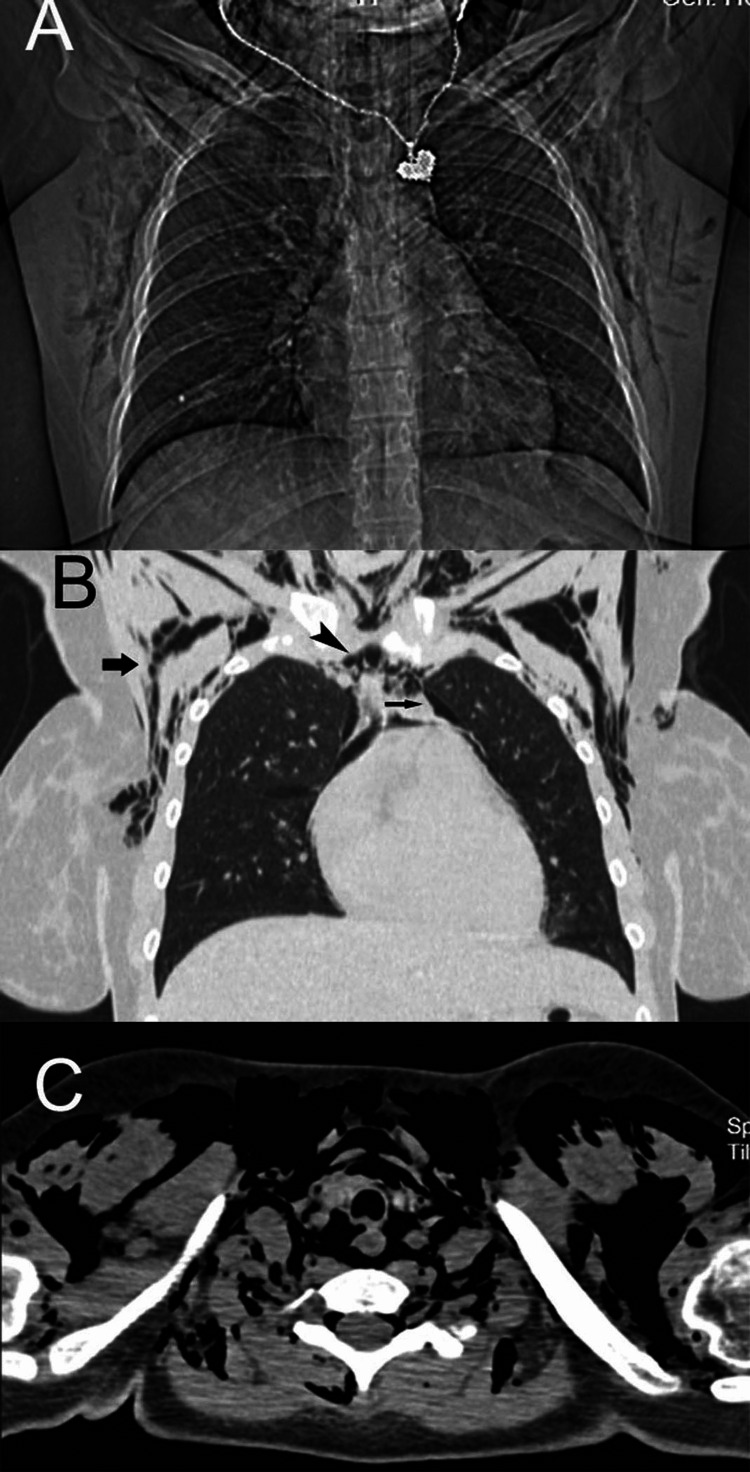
Scans of an 18-year-old female who developed pneumomediastinum, pneumothorax (arrow), pneumorrhachis, and subcutaneous emphysema with paroxysmal cough A: An anterior-posterior chest radiograph shows extensive soft tissue emphysema and a possible pneumothorax; B: A coronal reformatted CT image reveals pneumothorax (thin arrow), pneumomediastinum (arrowhead), and soft tissue emphysema (arrow) in the axillary regions and the neck; C: The axial CT image displays extensive emphysema. Free air resides in soft tissue, the mediastinum, and the spinal canal.
